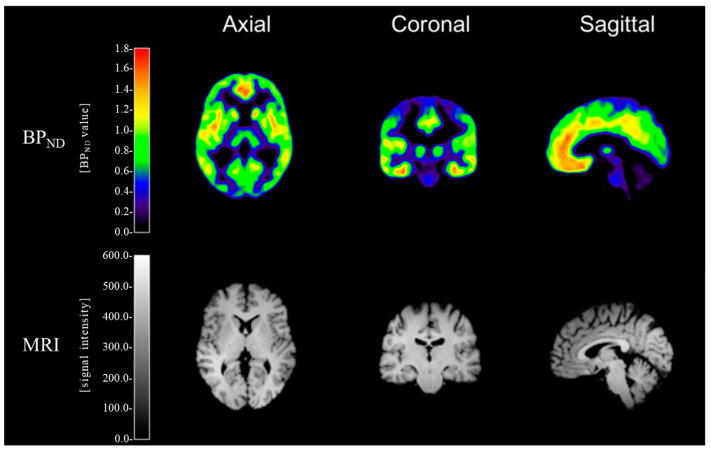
Representative examples of [^11^C]ABP688 BP_ND_ and 3-Tesla MR images of a control subject. (MNI coordinates: x = 3.0 mm, y = -19.5 mm, and z = 4.5 mm).
